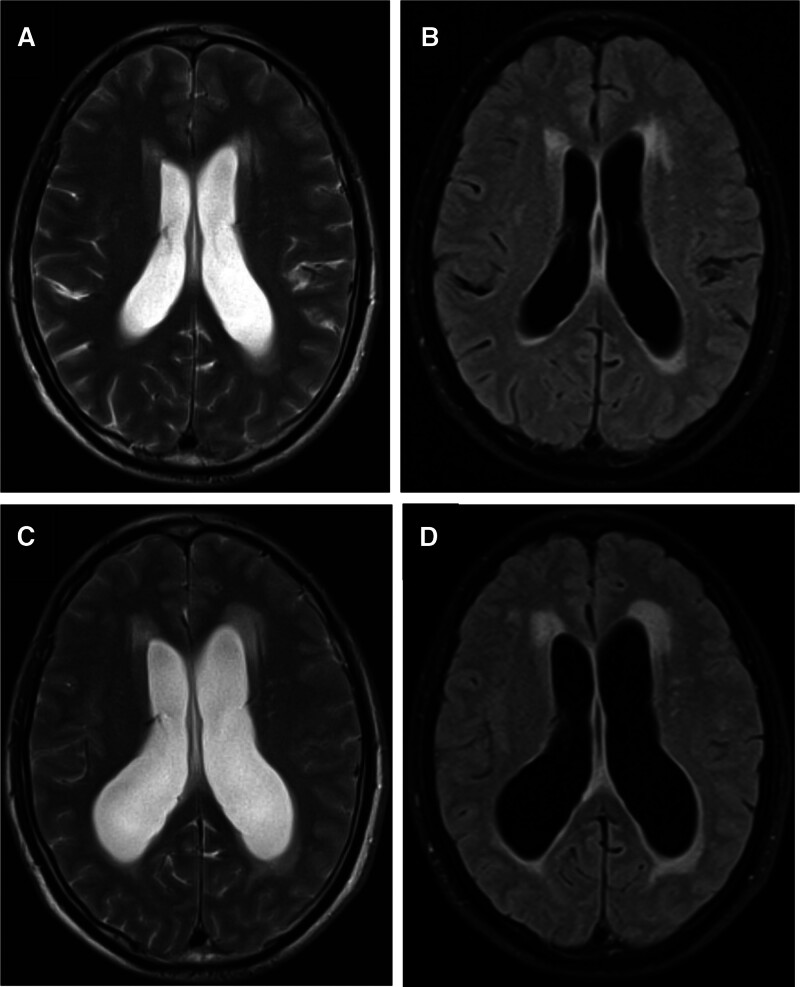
Cranial magnetic resonance imaging (MRI) of the patient. (A and B) Images of January 2018; (C and D) images of December 2019. (A) and (C) T2WI; (B) and (D) T2WI-Flair. (A and B) Cranial MRI images of the patient in 2018 showed mild hydrocephalus and a few high-signal intensities in the periventricular white matter. (C and D) Cranial MRI images of the patient in 2019 demonstrated progression of hydrocephalus. Compared to 2018 and an increase in the number of high-intensity signals within the cranium.
